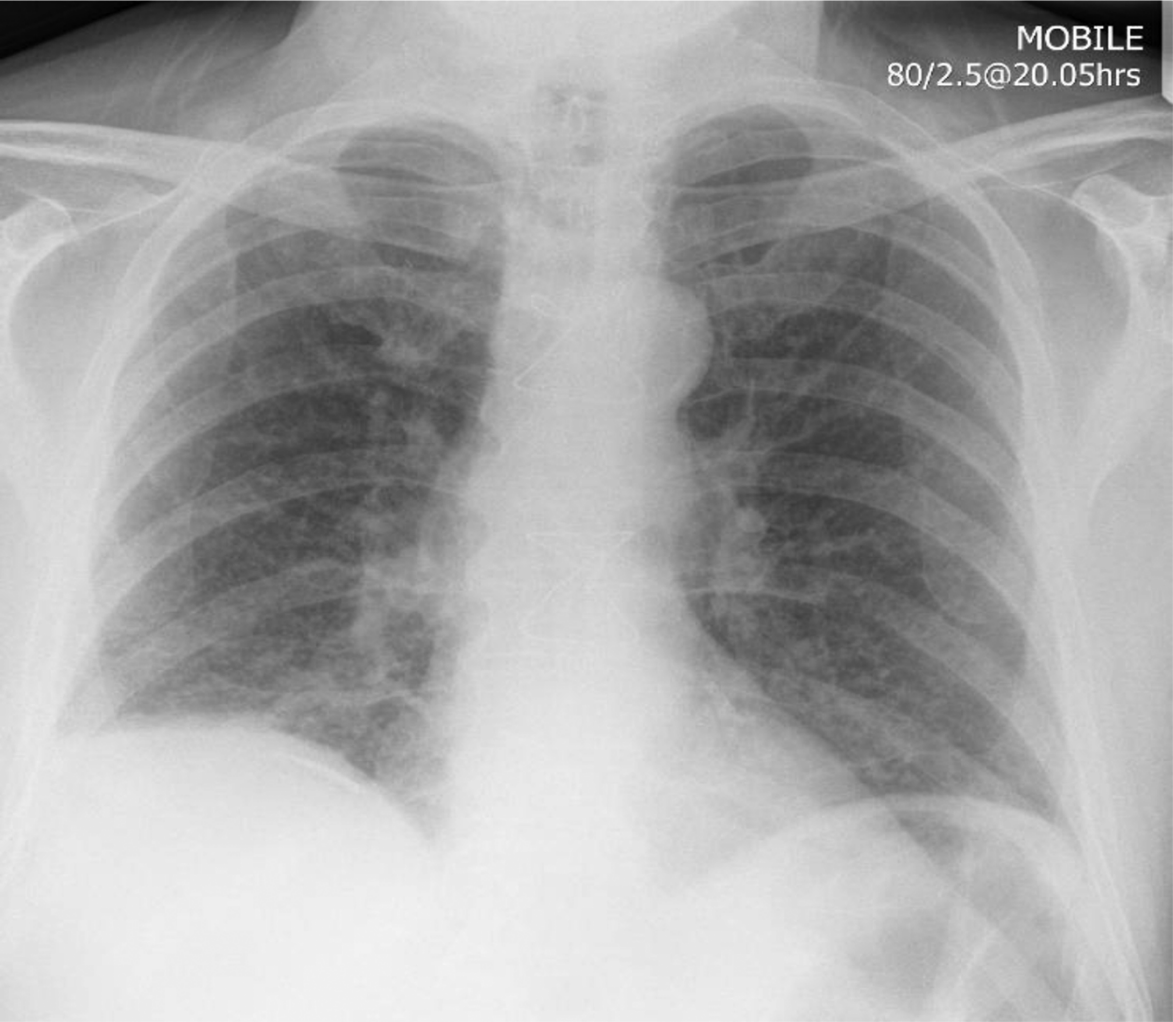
Anteroposterior erect chest X-ray showing fine micronodular shadowing.
